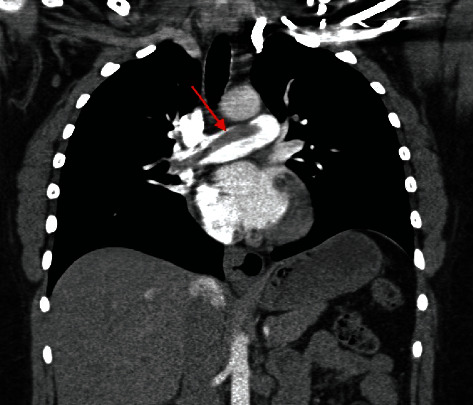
Computed tomography angiography coronal view demonstrated a large saddle embolism as shown by the red arrow.
